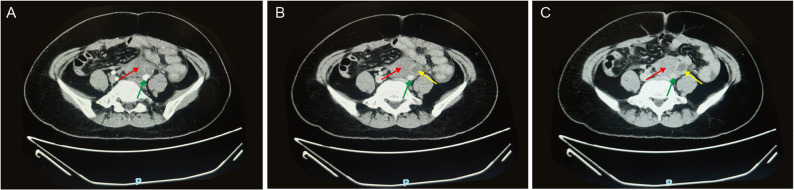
Representative computed tomography images of patients with Intra-abdominal aggressive fibromatosis. Computed tomography revealed a high-density mass (red arrowhead) located in front of the left lumbar psoas muscle, and the left iliac artery (green arrowhead) and left ureter (yellow arrowhead) were completely enveloped by the mass. A Enhanced arterial phase. B Enhanced venous phase. C Enhanced balanced phase
